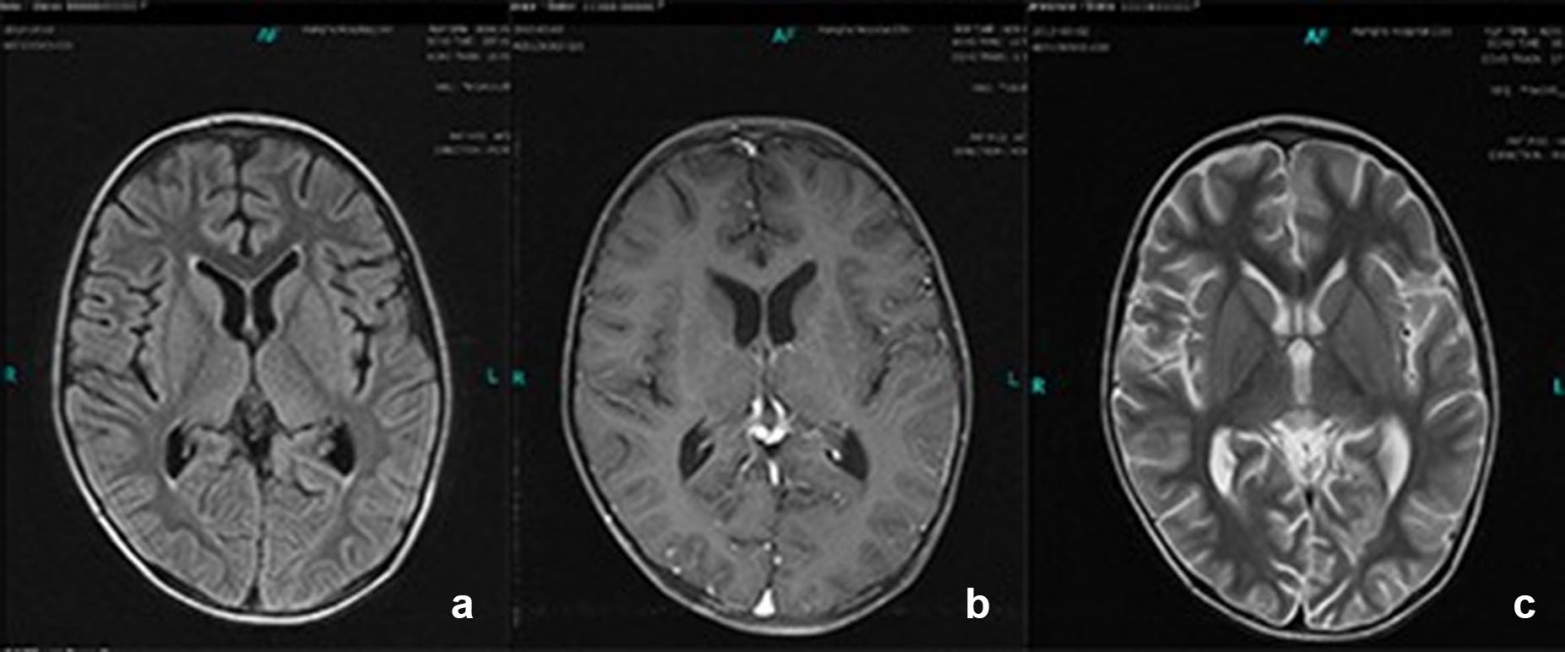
Head magnetic resonance imaging of case 5. Multiple spotted signals were seen in the bilateral white matter of the ventricle, and obvious enhancement was observed. The ventricular system was dilated.
